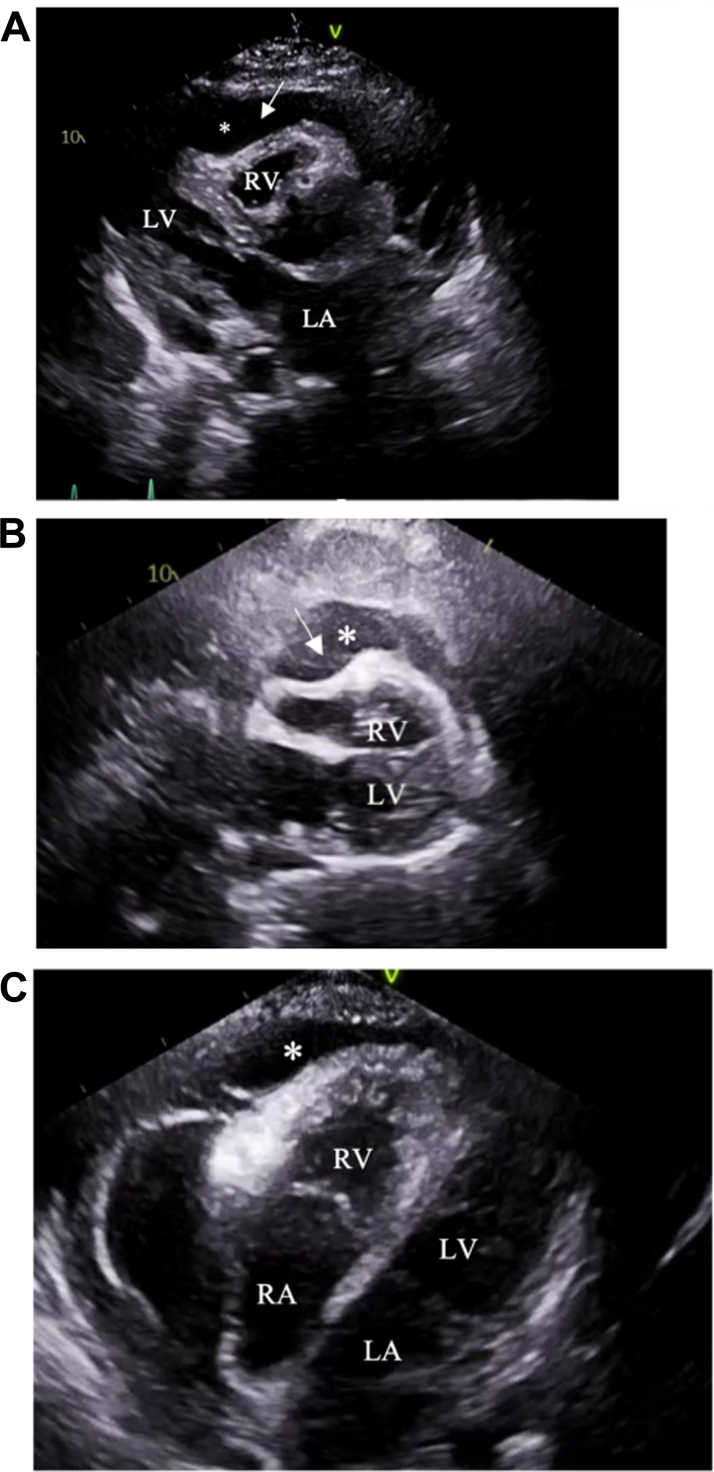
Transthoracic Echocardiography Showing Recurrent Pericardial Effusion and Tamponade (A) Parasternal long-axis view demonstrating large pericardial effusion and right ventricular (RV) collapse. (B) Subxiphoid view demonstrating large pericardial effusion and RV collapse. (C) Apical 4-chamber view with off-axis orientation demonstrating large pericardial effusion. Arrow indicates RV collapse. ∗Pericardial effusion. LA = left atrium; LV = left ventricle; RA = right atrium.
